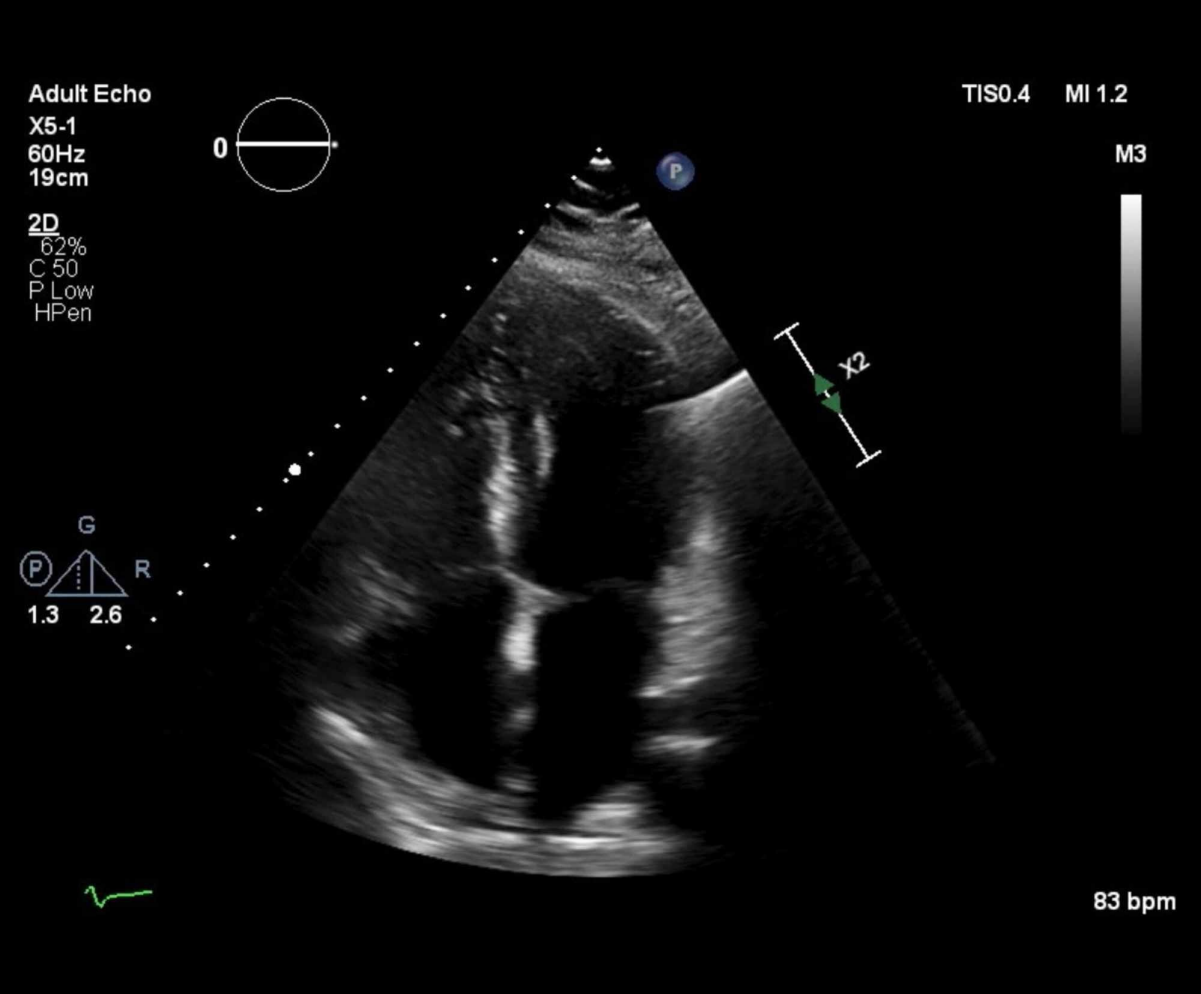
Apical four chamber view at one month post discharge Apical four chamber view on transthoracic echocardiogram (TTE) demonstrates resolution of pericardial effusion one month following discharge.
